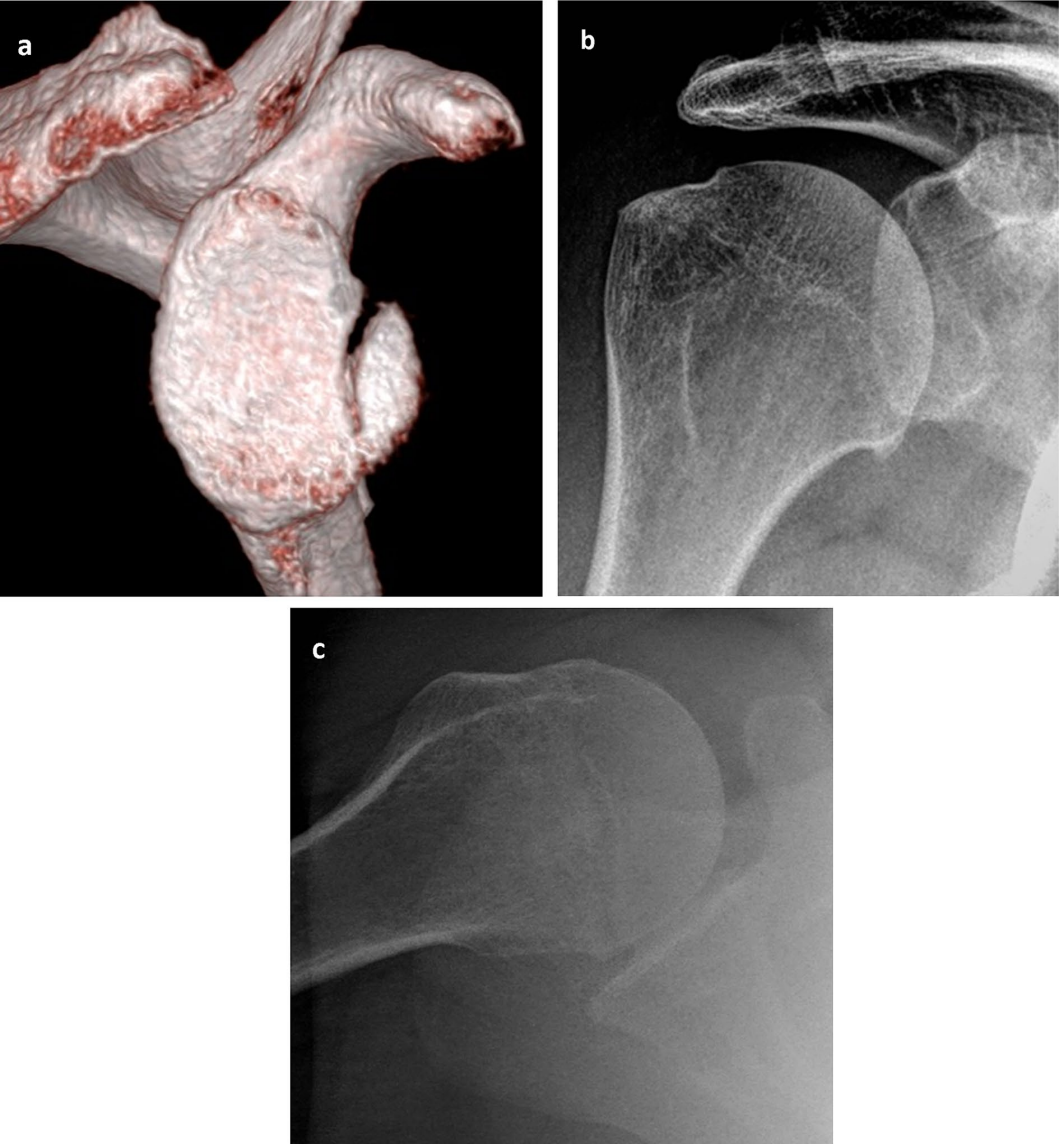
48-year-old patient with a minimally displaced non-operatively treated GRF (a 3D-CT, enface view) presented moderate OA (b A.P.-radiograph) 4 years later without reinstability and a free ROM (b, c A.P. and axial-radiograph)
